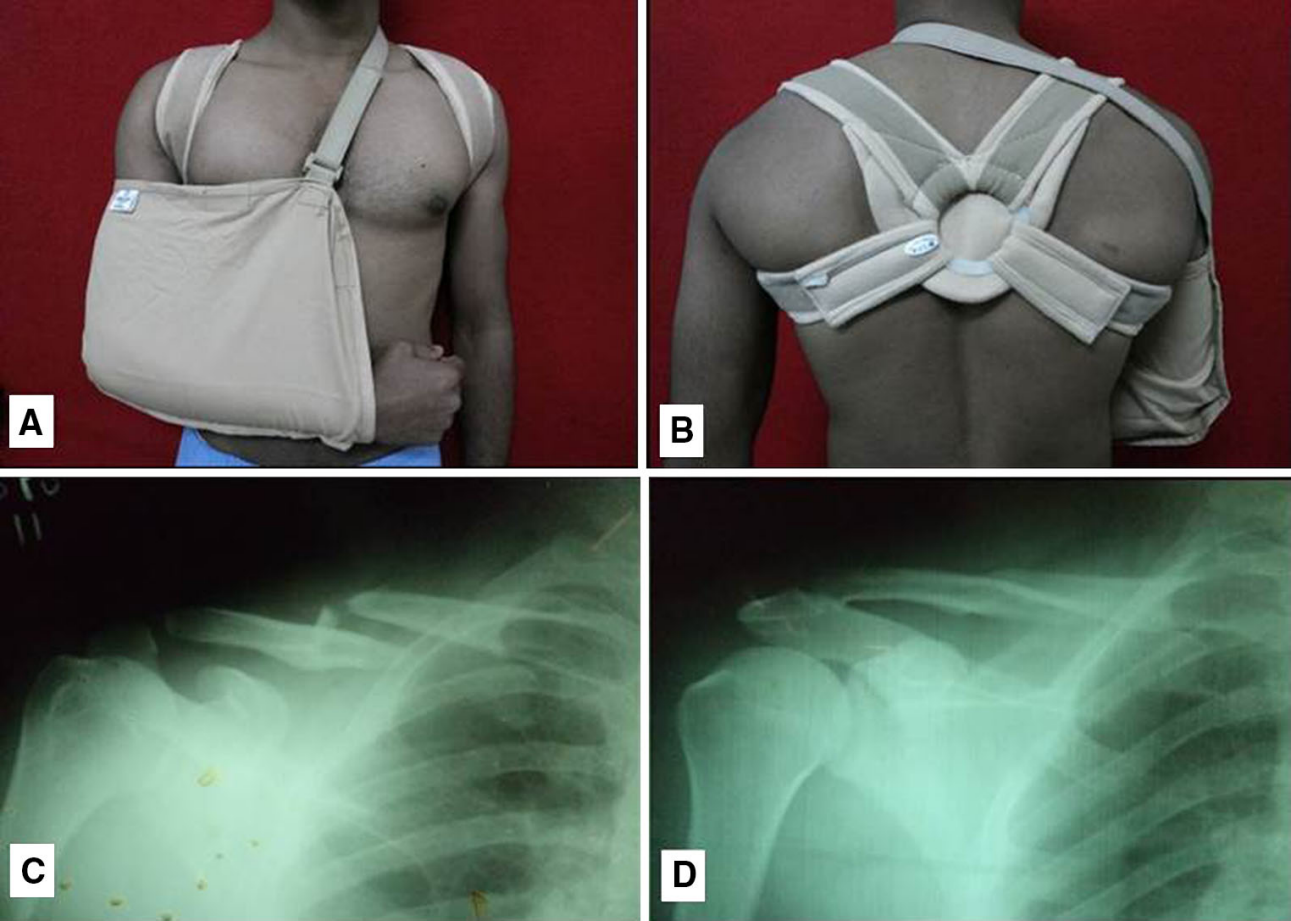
a Figure-of-eight bandage with shoulder arm pouch-anterior view. b Figure-of-eight bandage with shoulder arm pouch-posterior view. c Initial radiograph of the fracture at presentation. d Fracture union after 6 months of conservative treatment
